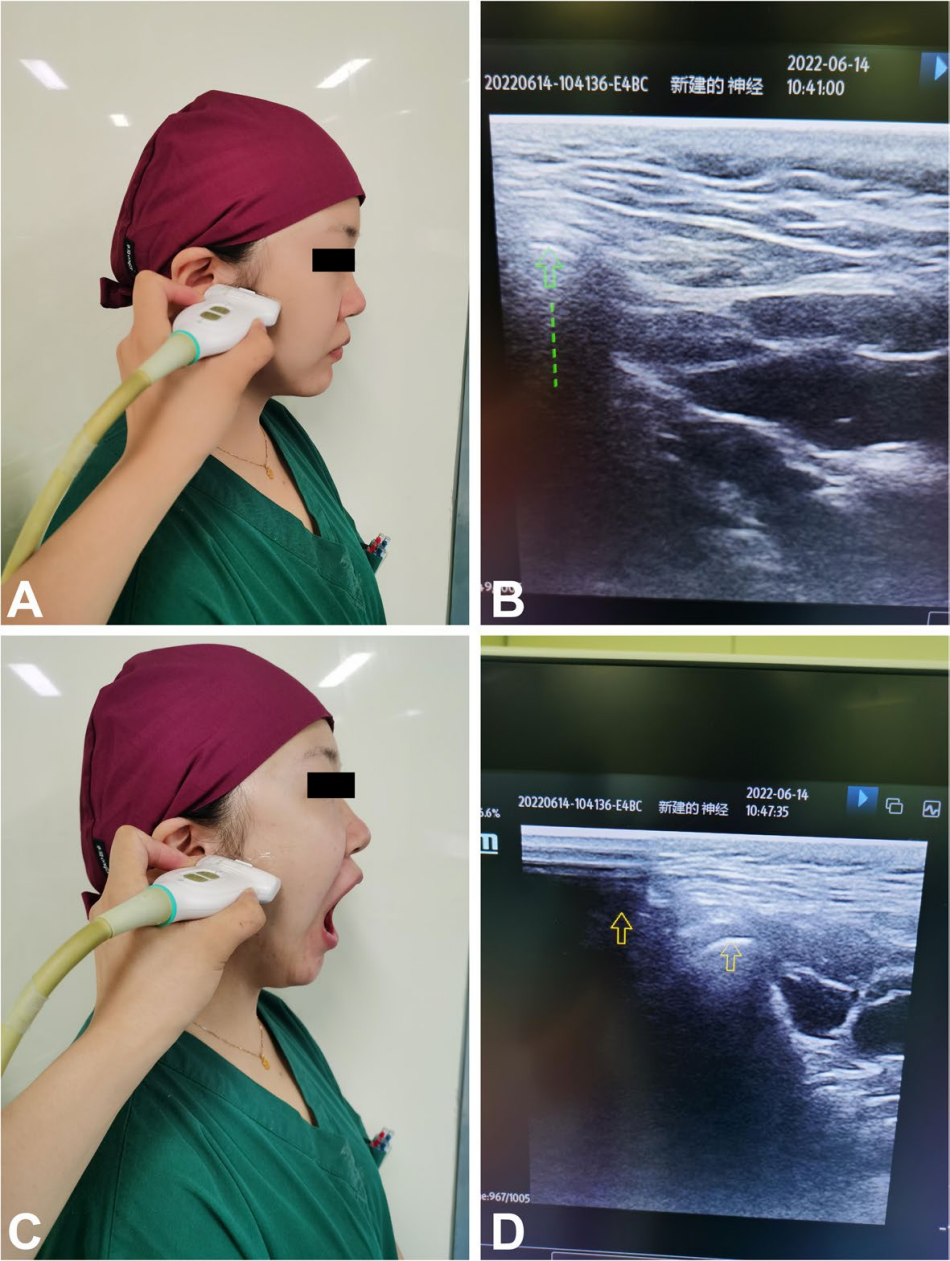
Temporomandibular joint mobility sonographic methods and condylar translation measurement. Ultrasound probe position (A and C) and images (B and D) captured separately when the mouth was opened and closed. When the 2 images were compared, the mandibular condyle position was shifted from one point (crosshair marked “1” in B and D) to the other (the other crosshair in D). The mandibular condylar mobility is 11.10 mm (D12)
